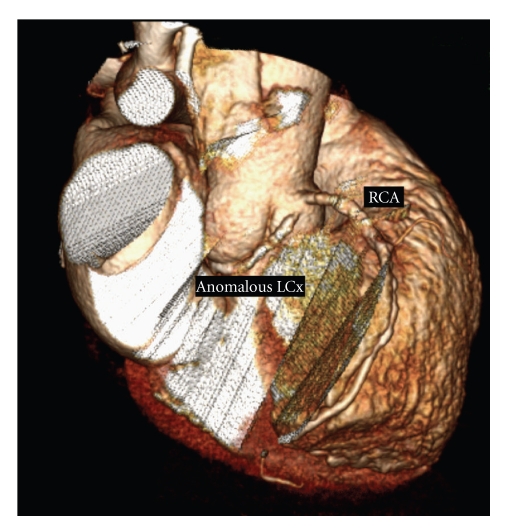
Angio CT showing retro-aortal route of anomalous LCx.
